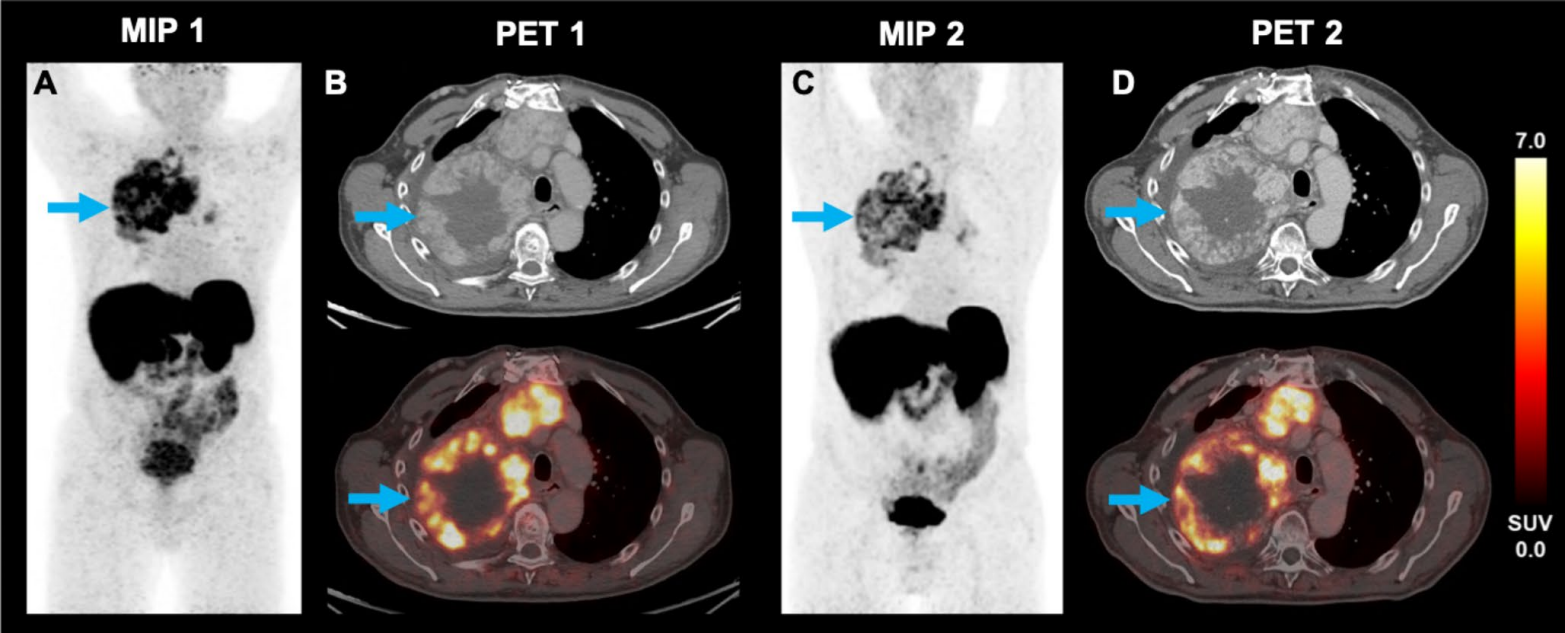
A 74-year-old male patient diagnosed with oncocytic carcinoma of the thyroid (formerly Hürthle cell variant FTC), who underwent iodine radioactive therapy and radiotherapy, was referred to our department due to a high thyroglobulin level of 606 ng/mL. The maximum intensity projection (MIP) and selected transversal levels of a mediastinal tumor bulk (blue arrow) of the baseline and follow-up PET are shown. The baseline [^18^F]SiTATE PET/CT (A-B) revealed a TTV of 385 mL. Metastases were located mediastinal, bipulmonary and hilar. After two cycles of [^177^Lu]Lu-DOTA-TATE therapy the thyroglobulin level was 273 ng/mL and [^18^F]SiTATE PET/CT showed a TTV of 273 mL (C-D), suggestive for partial response
